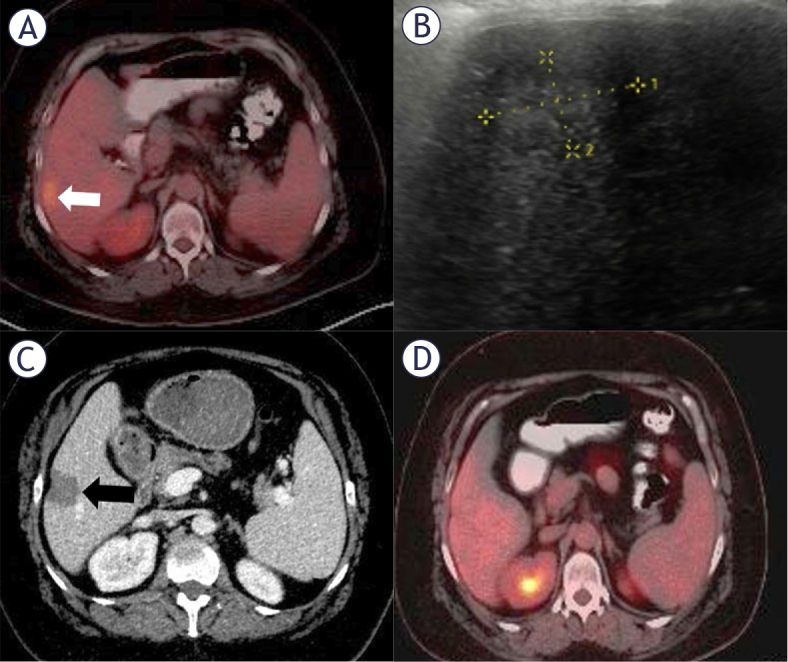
(A-D) Radiological findings in a 48-year-old woman with ductal breast carcinoma. PET-CT revealed a hypermetabolic metastasis in segment 6, (A) (white arrow). Ultrasound showed a subcapsular hypoechoic lesion (B), which was treated with percutaneous microwave ablation under ultrasound guidance. Follow-up CT one day after ablation demonstrated a lesion/ablation area ratio of 1.6 without contrast enhancement, (C) (black arrow). PET-CT at 36 months showed no pathological FDG uptake in the liver (D).
